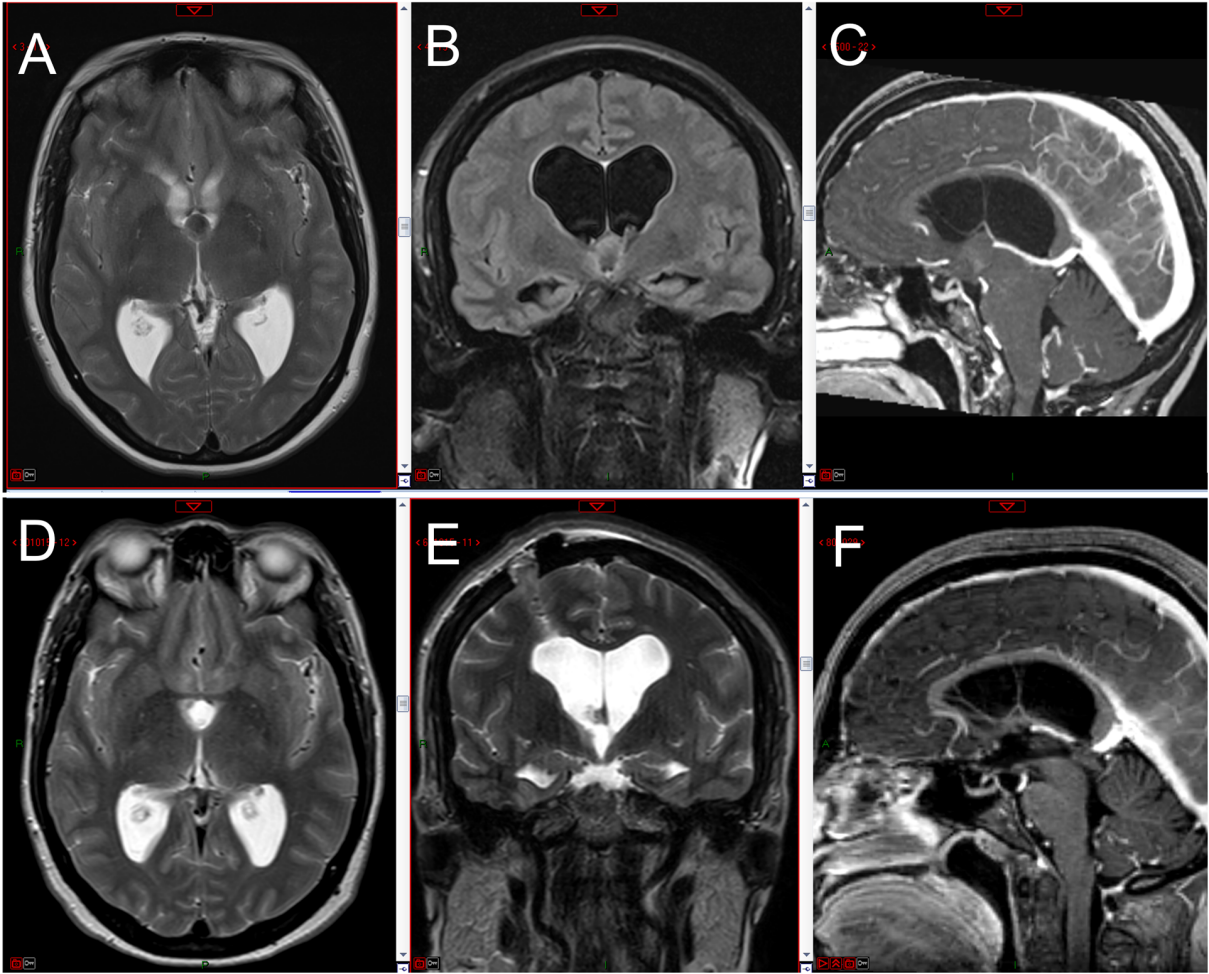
Preoperative MRI images (axial T2-weighted, coronal FLAIR T2-weighted, sagittal enhanced T1-weighted) showing the third ventricle colloid cyst with bilateral ventriculomegaly (A–C); 48 h postoperative MRI images showing complete removal of the cyst with initial reduction in ventricular size (D–F).
